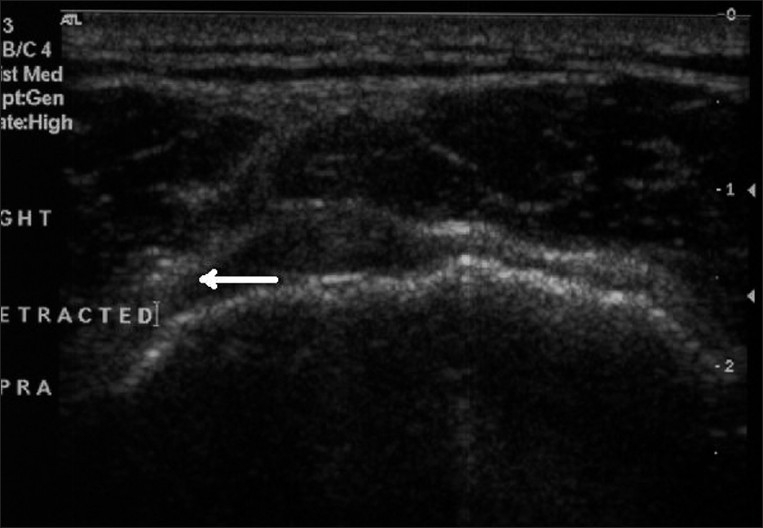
Ultrasound showing full-thickness retracted tear in the right supraspinatus tendon (arrow)
